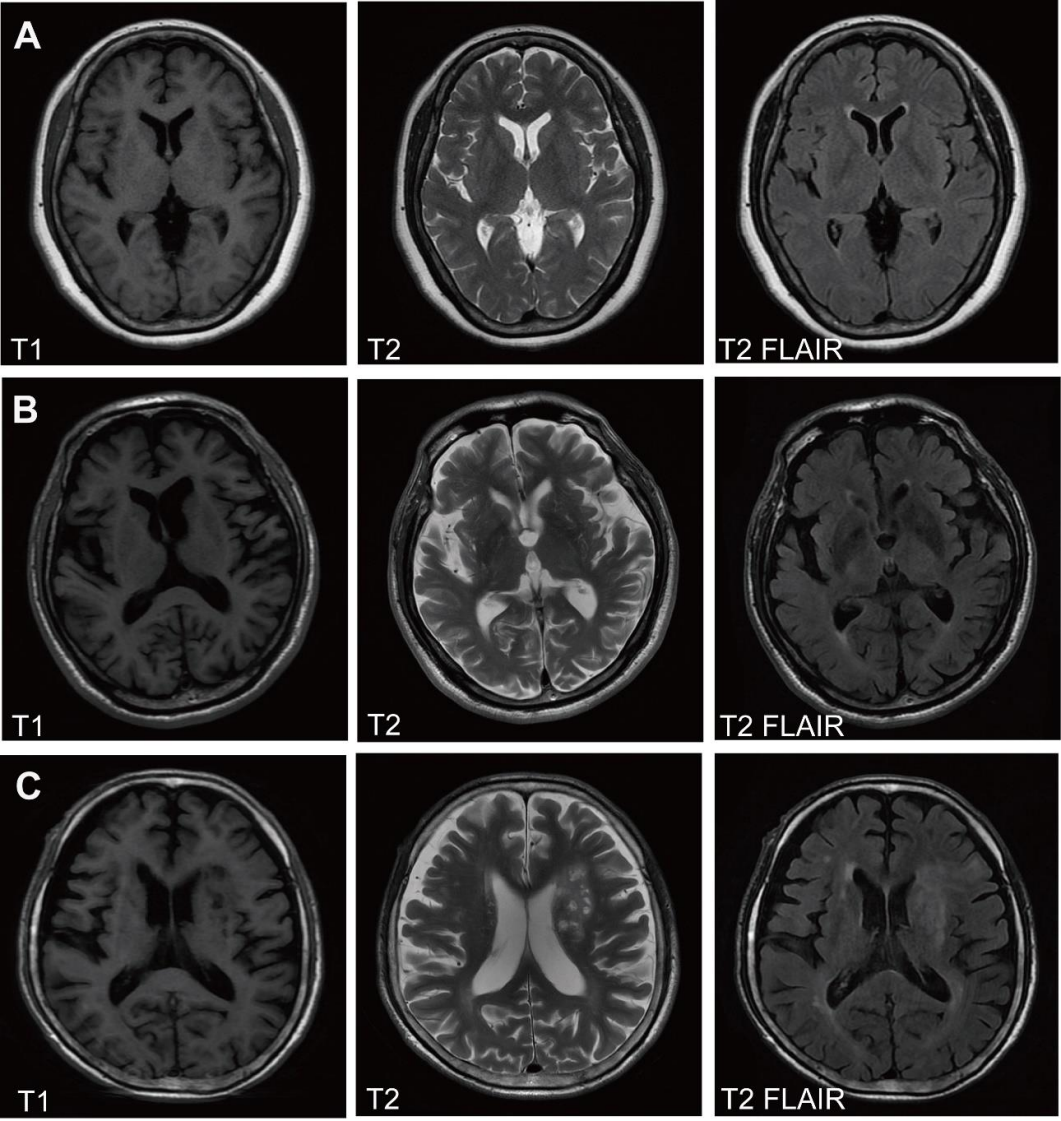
MRI images in normal controls and PDD and VPD patients (A) Normal subjects, (B) PDD patients, (C) VPD patients. The extent of white matter hyperintensities and multiple infarctions in the basal ganglia in the VPD patients are shown in T2-weighted and FLAIR images. Arrows indicate the infarction.
